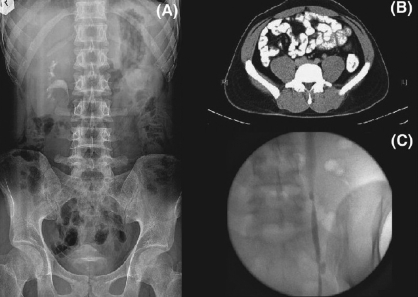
(A) IVU showing a poorly visualized left renal pelvis and a non-visualized left ureter. (B) CT scan showing a left ureteric stricture, which was no recognized in the IVU. (C) Retrograde ureterogram showing multiple upper and mid-ureteric strctures
